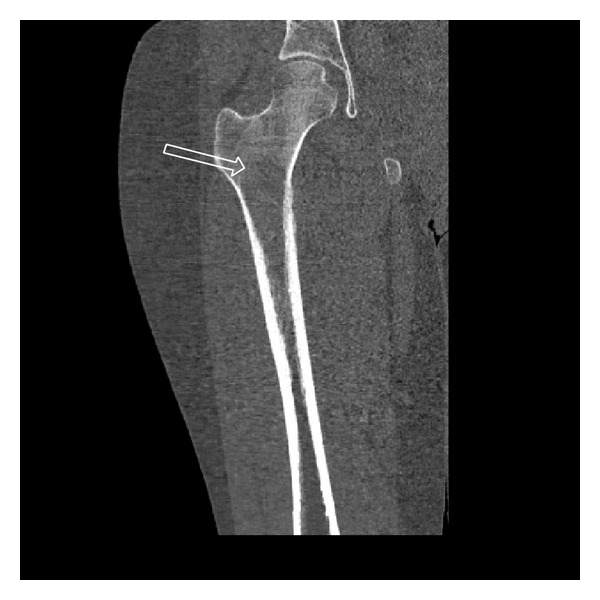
Coronal CT reformation of the right proximal femur reveals subtle low-attenuating lesion without cortical destruction or soft-tissue component. The arrow points to the superior margin of the ill-defined lesion.
